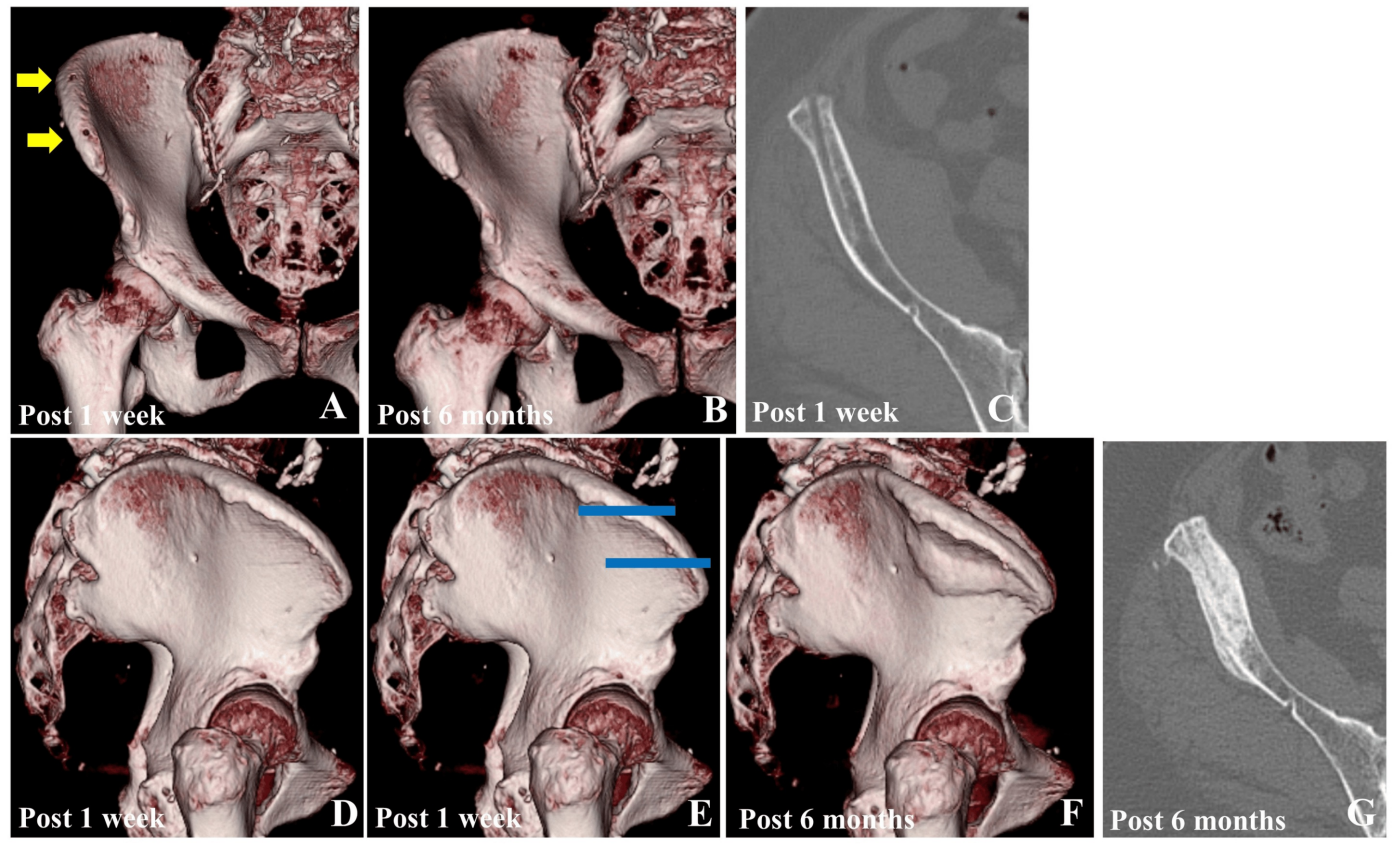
Postoperative 3D and CT images of case two A: The 3D image of the pelvis one week postoperatively. The yellow arrows indicate the insertion sites of two bone pins. B: The 3D pelvic image at six months postoperatively shows the bony union of the inner cortical layer of the ilium without displacement. C: Axial CT image of the pelvis at the level of the distal pin hole at one week postoperatively, demonstrating long transcortical fixation through the outer cortex. D: The 3D lateral pelvic image at one week postoperatively shows no visible pin holes in the outer cortex of the ilium. E: Lateral pelvic image at one week postoperatively, with an overlay of the bone pin insertion sites indicated by blue lines. F: Lateral pelvic image at six months postoperatively. The fracture line extended along the line of transcortical fixation. G: Axial CT image of the pelvis at the level of the distal pinhole six weeks postoperatively, showing the bony union of the iliac bone.
